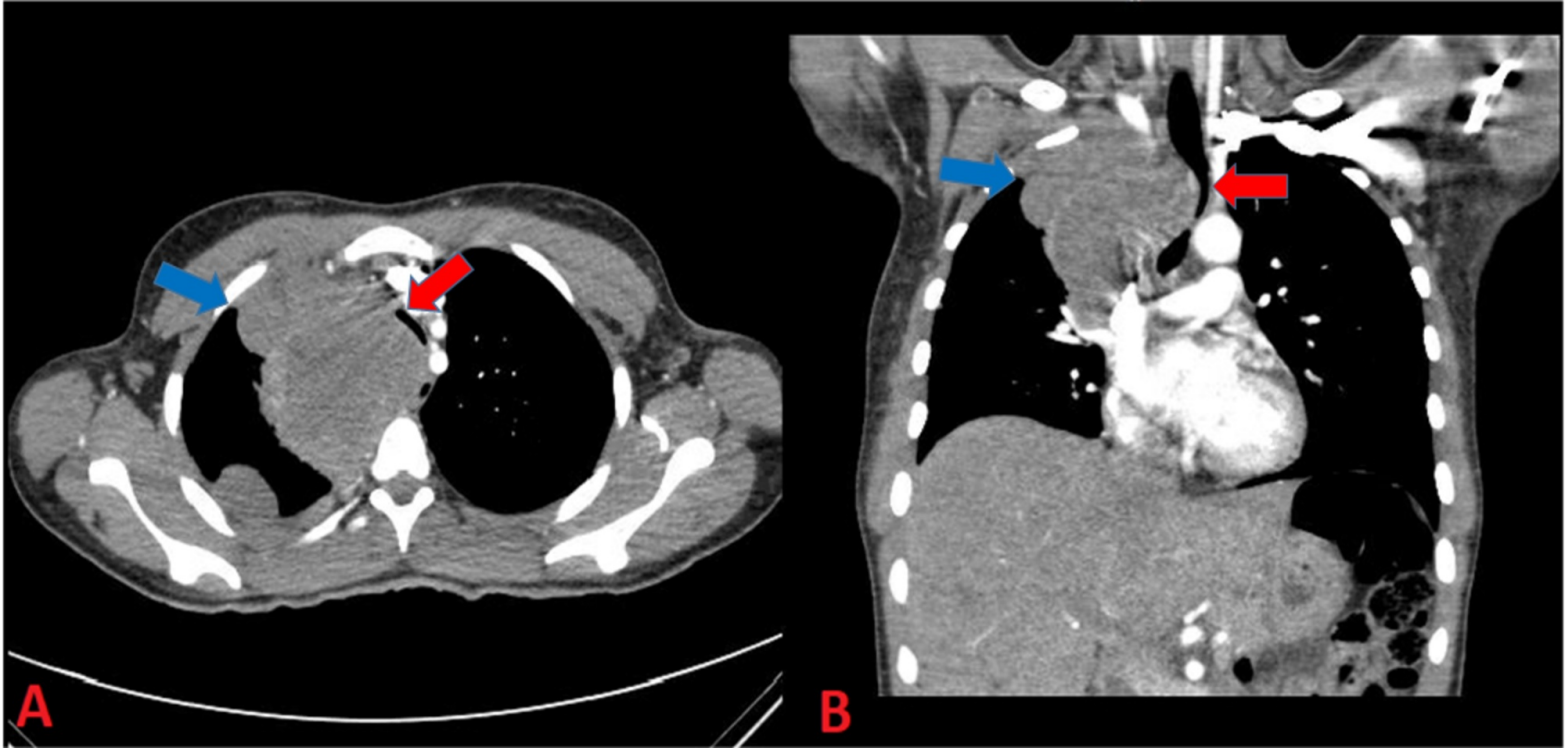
Computed tomography angiography (CTA) of the chest (axial and sagittal view) 1A. CT scan of chest showing a heterogeneous superior right mediastinal mass measuring 10 cm craniocaudally and a 3.6 cm mass in the right middle lobe (blue arrow) and severe tracheal stenosis (red arrow). 1B. CT scan of chest showing lung mass (blue arrow) compression leading to “coin slit” trachea (red arrow).
